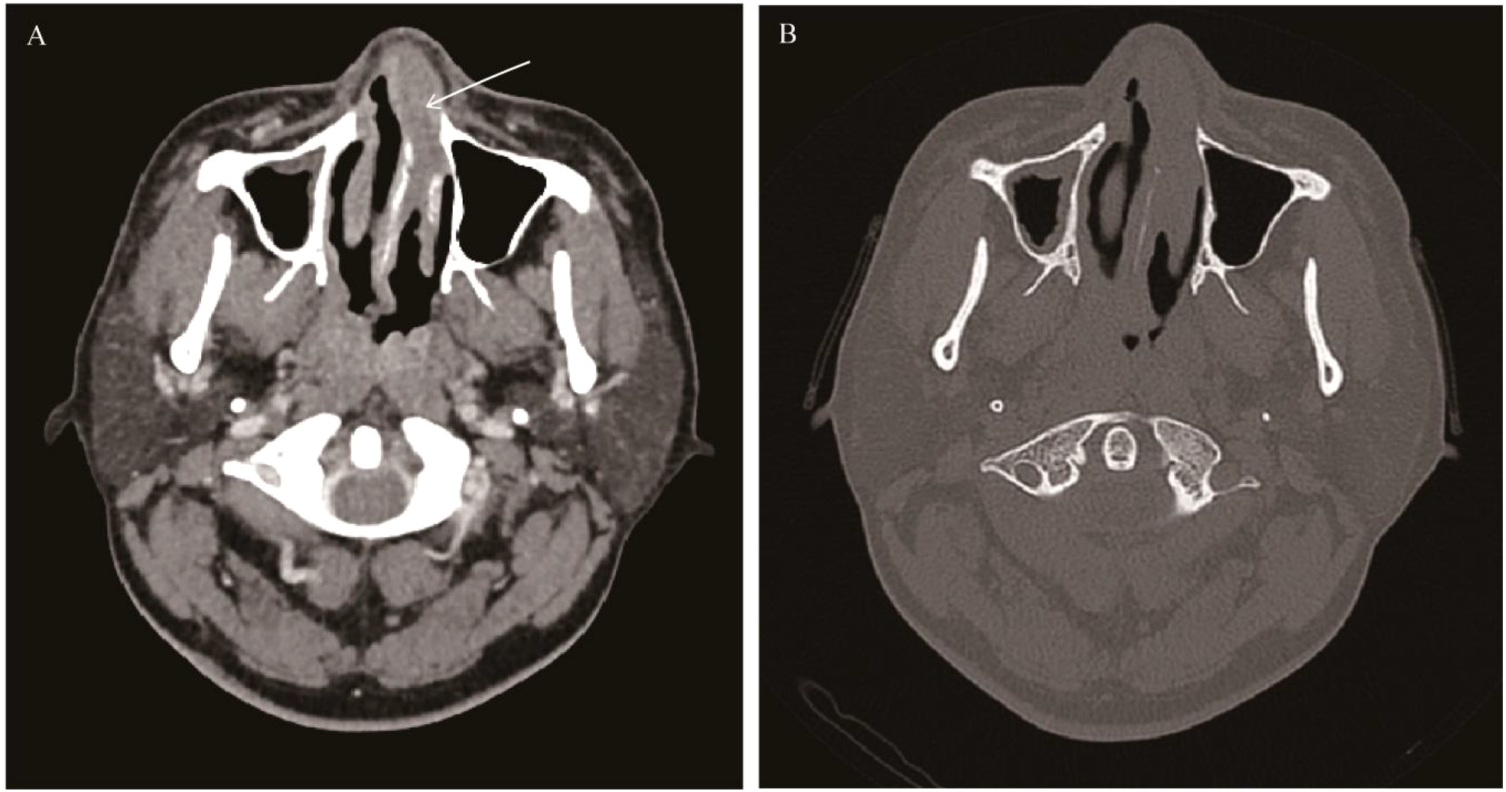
NK/T-cell lymphoma: Axial CT showed soft tissue masse in the left nasal cavity and nasal vestibule (white arrow), with mild bone destruction and uniform enhancement of the masse (A, B).
